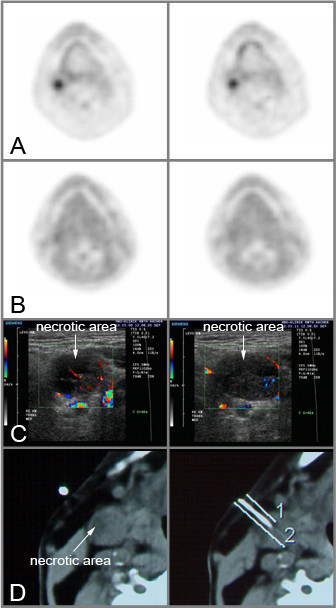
Outlier patient 4, transaxial FDG PET- (A), transaxial FMISO PET- (B), CDS- (C) and transaxial CT (D) scans (pO_2 _= 2.5 mmHg= 43.0%, pO_2 _= 5.0 mmHg = 53.0%, pO_2 _= 10.0 mmHg = 71.5%, median pO_2 _= 3.7 mmHg, mean pO_2 _= 8.8 mmHg; CPD = 2.96%; FDG_SUVmax _= 4.80, FDG_SUVmean _= 4.55; FMISO_T/M _= 1.19, FMISO_T/B _= 1.11). 1/2 = distance measurements for the guidance of the polarographic needle electrode.
